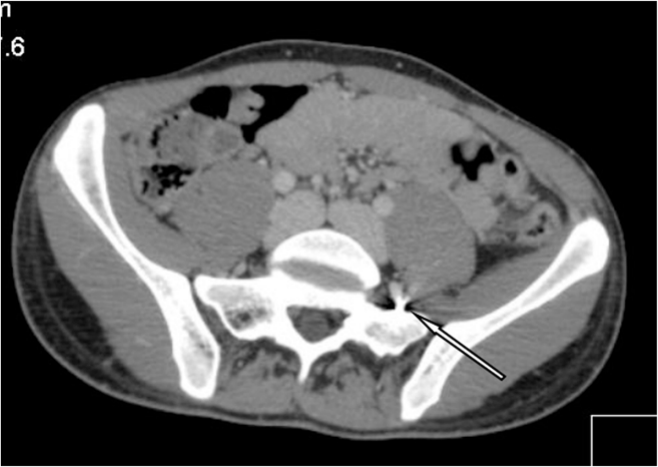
Axial post contrast CT image.
